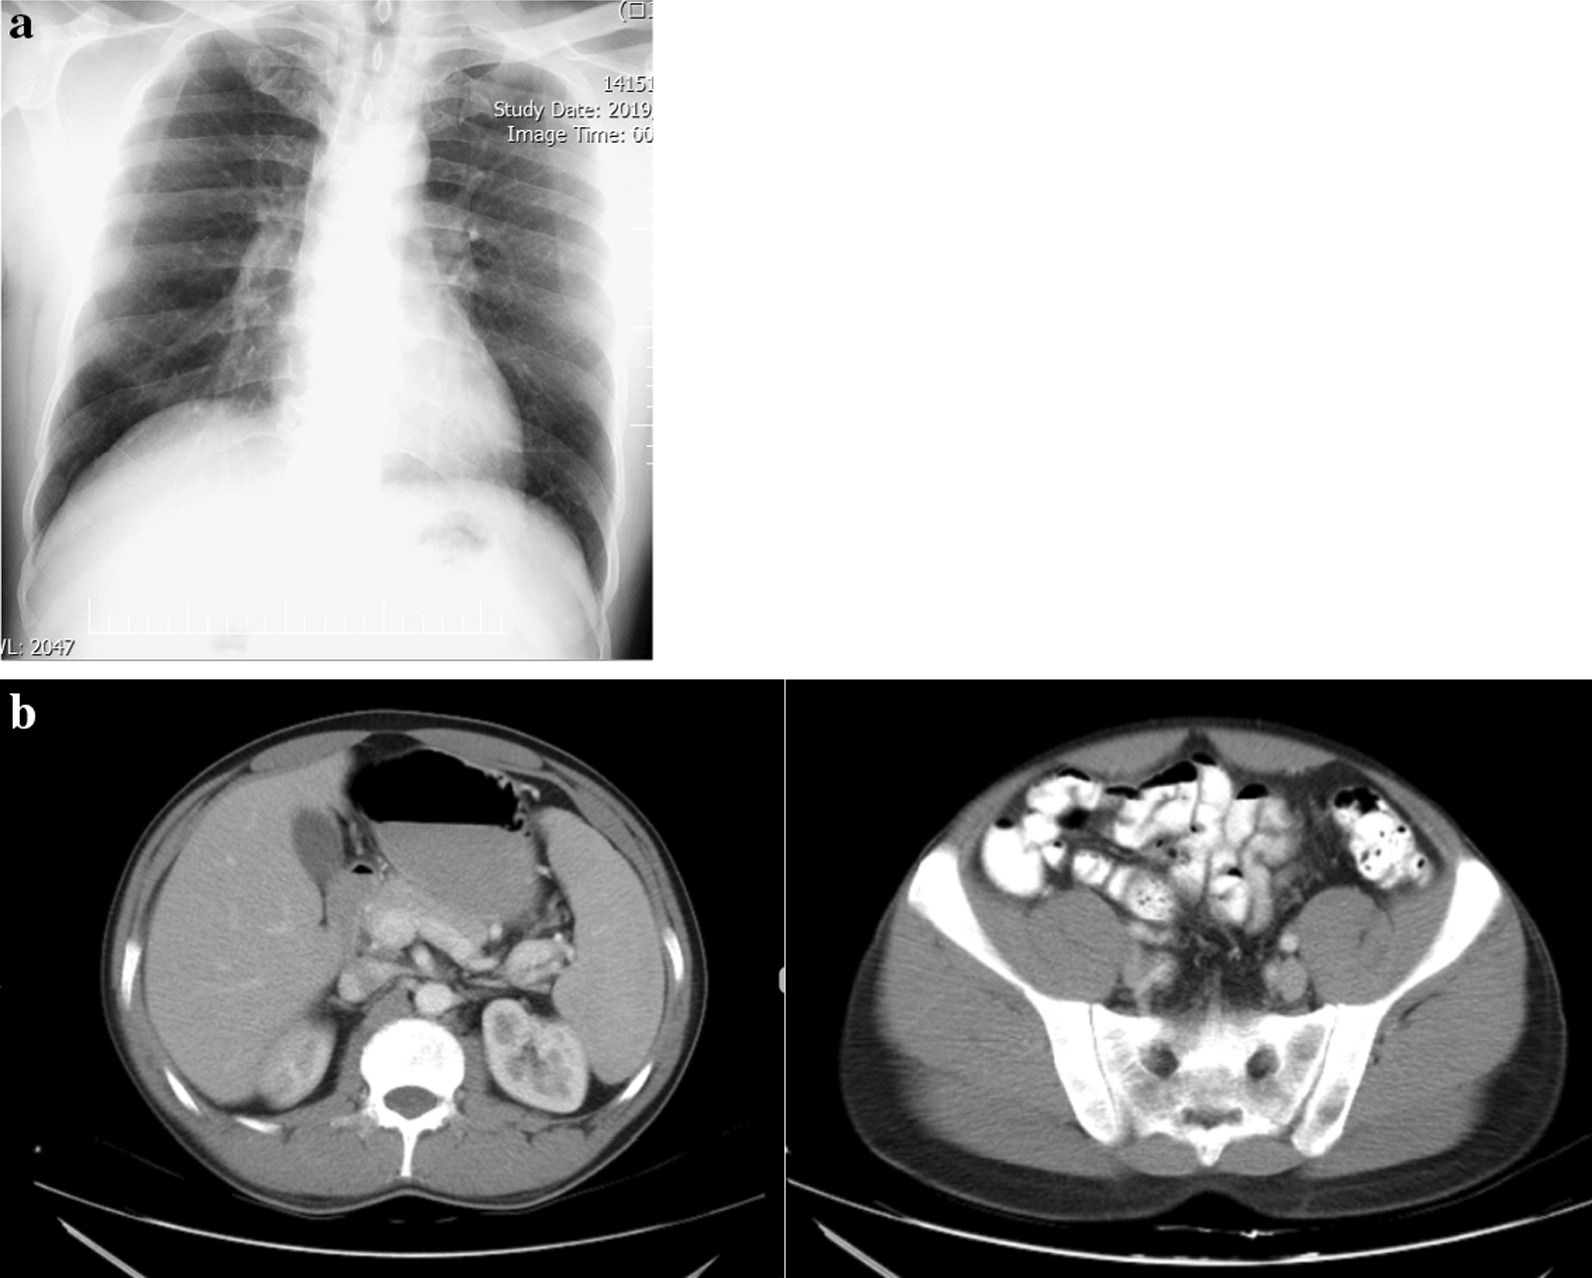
a Chest x-ray. b Computed tomography scan of the abdomen and pelvis showing no pathologic findings
